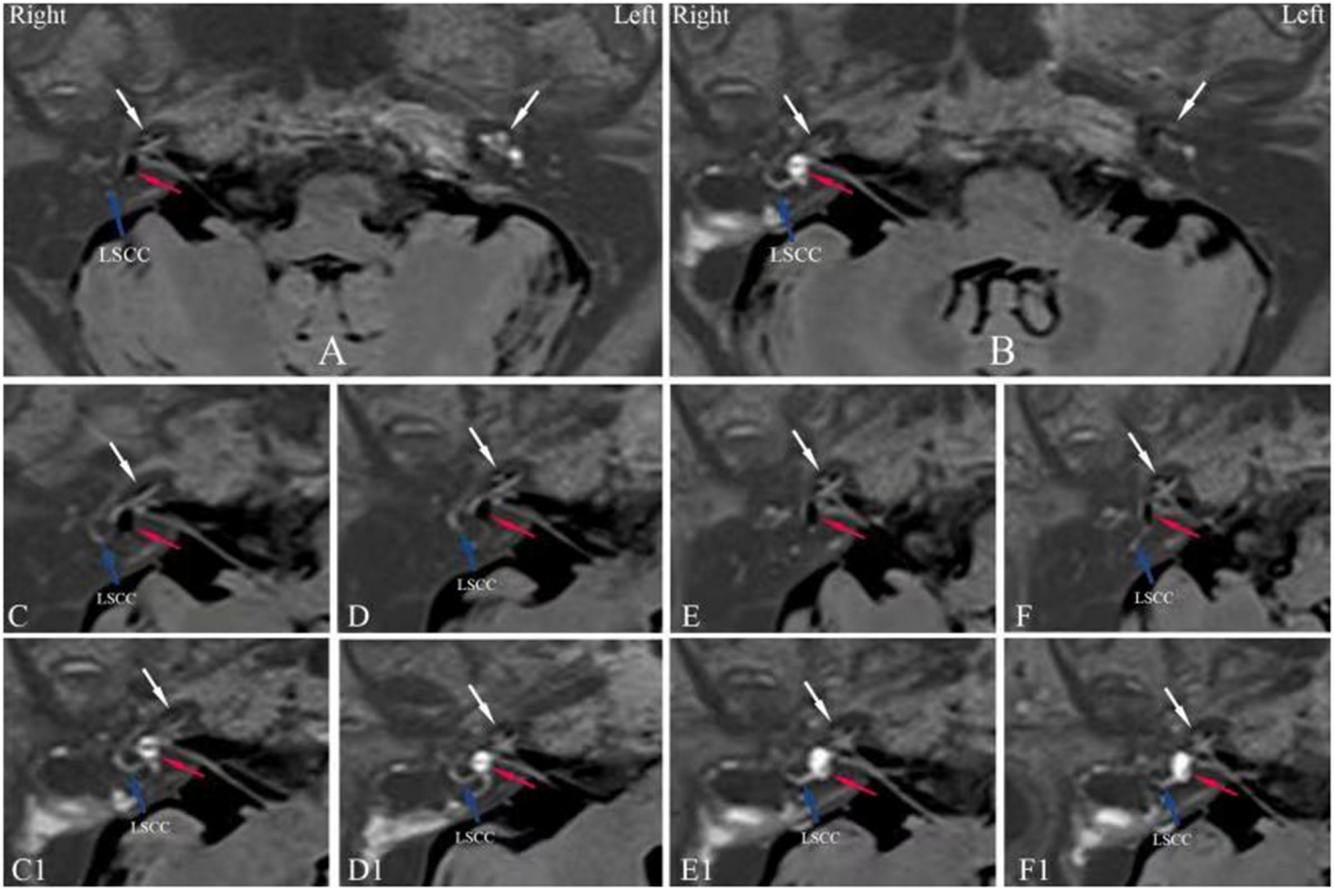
Axial IT-Gd+IV-Gd MRIs of patient no. 14 (Table 1) with right MD prior to surgery (A,C–F) and 16 months (B,C1–F1) after surgery. (A) An significant EH in the vestibule (red arrow) and cochlea (white arrow) in right ear and no signal void (endolymphatic space) with only enhanced perilymphatic space in left unaffected ear (white arrow). (B) The image shows complete reversal of vestibular hydrops and partial reversal of cochlear hydrops in the right ear in the same section plane as in (A), where the low-signal area of the vestibule shown in (A) has changed to a high-signal area (red arrow) and a significant cochlear EH has changed to a mild EH (white arrow). Whereas, an axial IV-Gd MR image of the left unaffected ear shows insufficient contrast in cochlea (white arrow). The serial MR images in (C–F) show a significant EH in vestibule (red arrow) and cochlea (white arrow) before surgery. The serial MR images in (C1–F1) show a disappearance of vestibular EH (red arrow) and the reduction of cochlear EH (white arrow) 16 months after surgery. LSCC, lateral semicircular canal; 3D-real IR, three-dimensional real inversion recovery; MRI, magnetic resonance imaging; MD, Meniere's disease; EH, endolymphatic hydrops; IT-Gd, intratympanic injection of gadopentetate dimeglumine; IV-Gd, intravenous injection of gadopentetate dimeglumine.
